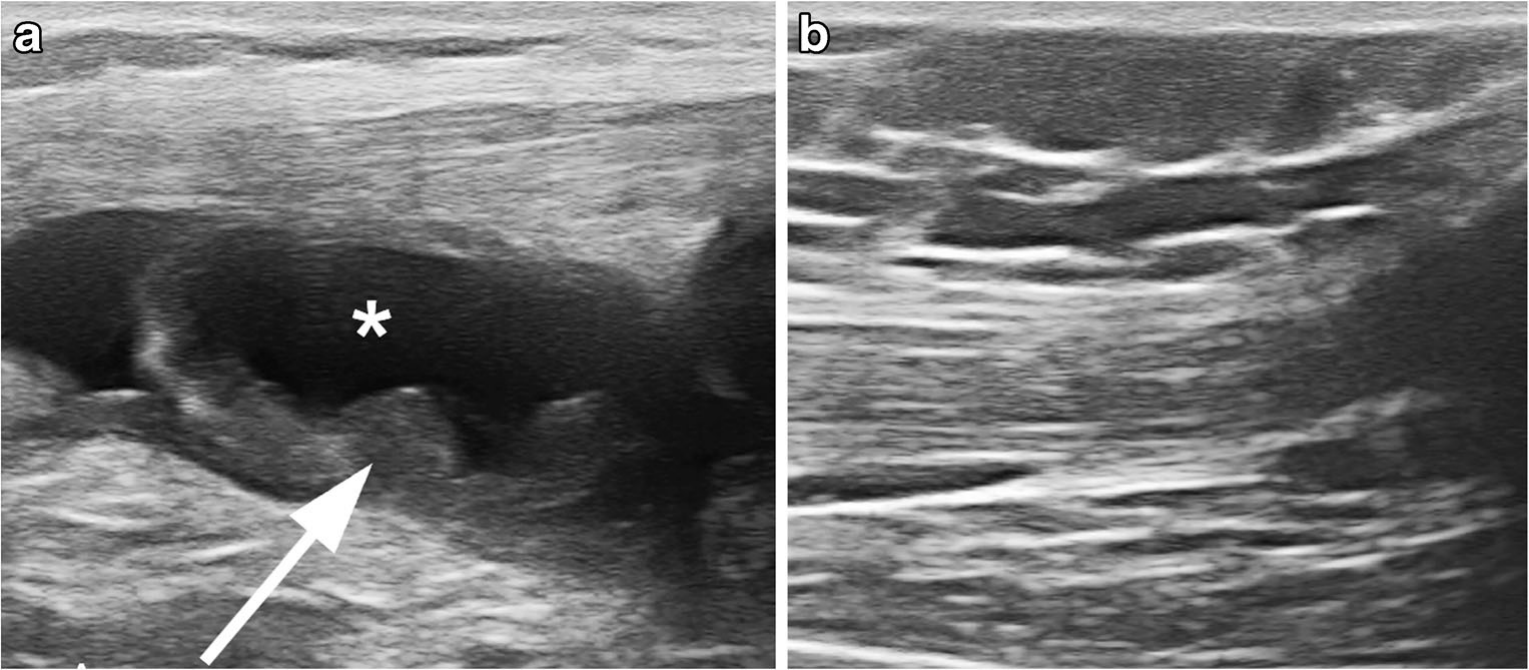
Ultrasound longitudinal to the suprapatellar recess in an 8-year-old girl with juvenile idiopathic arthritis. a There is evident synovial hypertrophy (*arrow*) and joint fluid in the suprapatellar recess (*). b After several months of treatment, the synovial hypertrophy and joint fluid have disappeared
